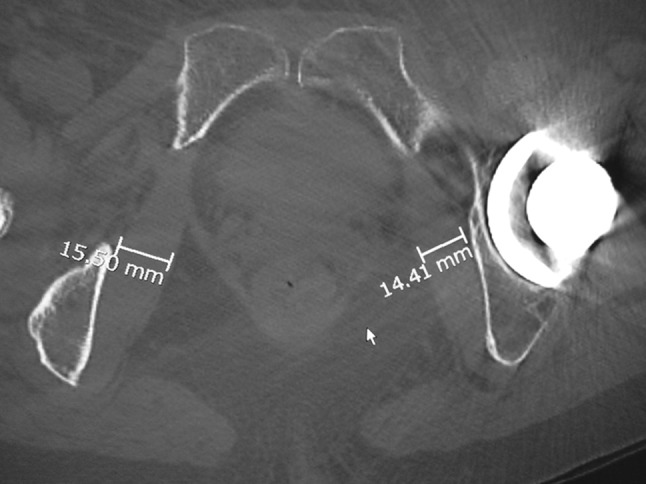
We calculated the maximum width of the internal obturator muscle belly on CT images of the affected and unaffected sides preoperatively, 10 days postoperatively, and 6 months postoperatively
